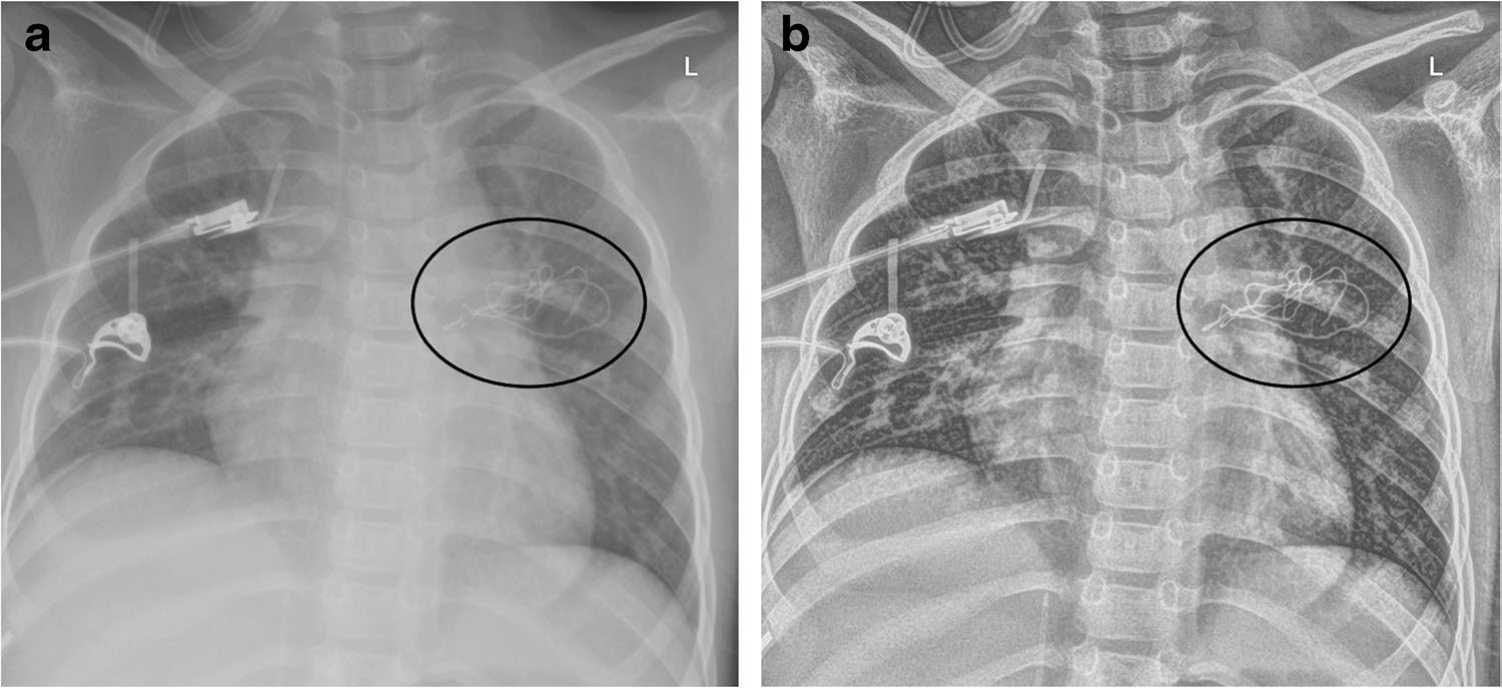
An anteroposterior chest radiograph (69.6 kV, 4.0 mAs, 3.91 cGy·cm^2^, exposure index: 376.78) unprocessed image (a) versus an S-Enhance image (b) in a 2-year-old girl (weight: 11 kg) with postinterventional foreign material (x-ray marker on compress) in projection to the left part of the chest (*black circle*)
